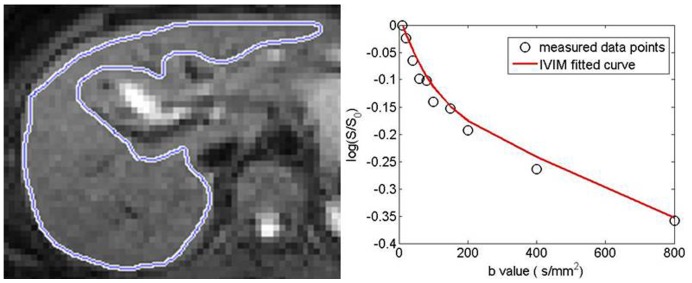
Region of interest (ROI) on the b = 0 diffusion weighted MR image (left) and the corresponding IVIM fitted curve of measured signal (right), showing a bi-exponential decay. The ROI was placed over the whole liver avoiding artifacts and blood.
